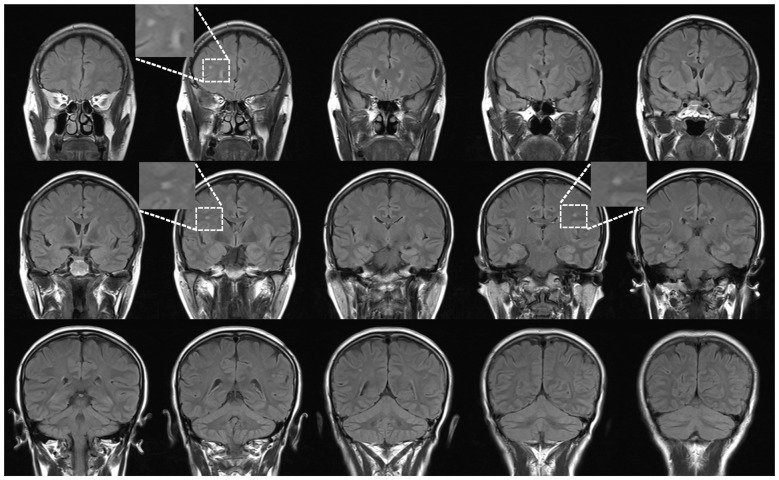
An example case: coronal view of fluid-attenuated inversion recovery (FLAIR) in a 46-year-old female subject. All participants with sporadic white matter hyperintense lesions underwent a health examination.
